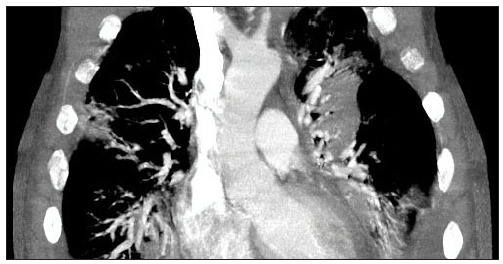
Thoracic computed tomography from the second report.
